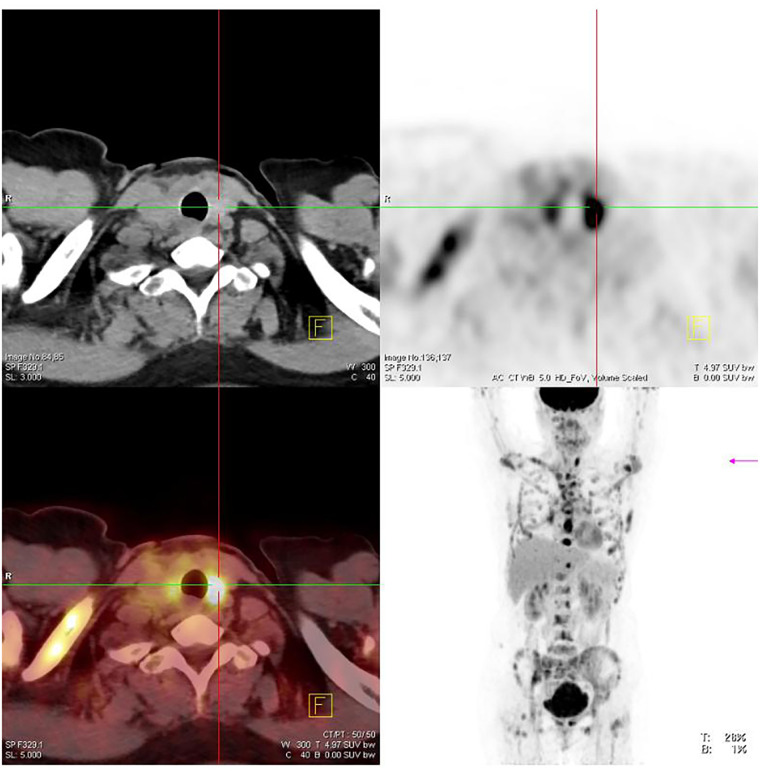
PET-CT indicated multiple systemic bone metastases.
